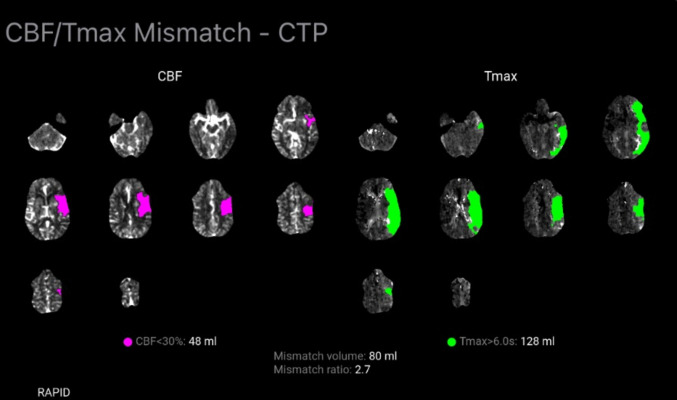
Computed tomography (CT) perfusion of the brain demonstrates significant mismatch volume secondary to a left middle cerebral artery (MCA) infarct. On the left images, brain areas with less than 30% cerebral blood flow are shown in purple; on the right panel, areas with Tmax more than 6.0 s are shown in green. Mismatch volume of 80 ml demonstrates considerable amount of salvageable brain tissue for endovascular intervention
